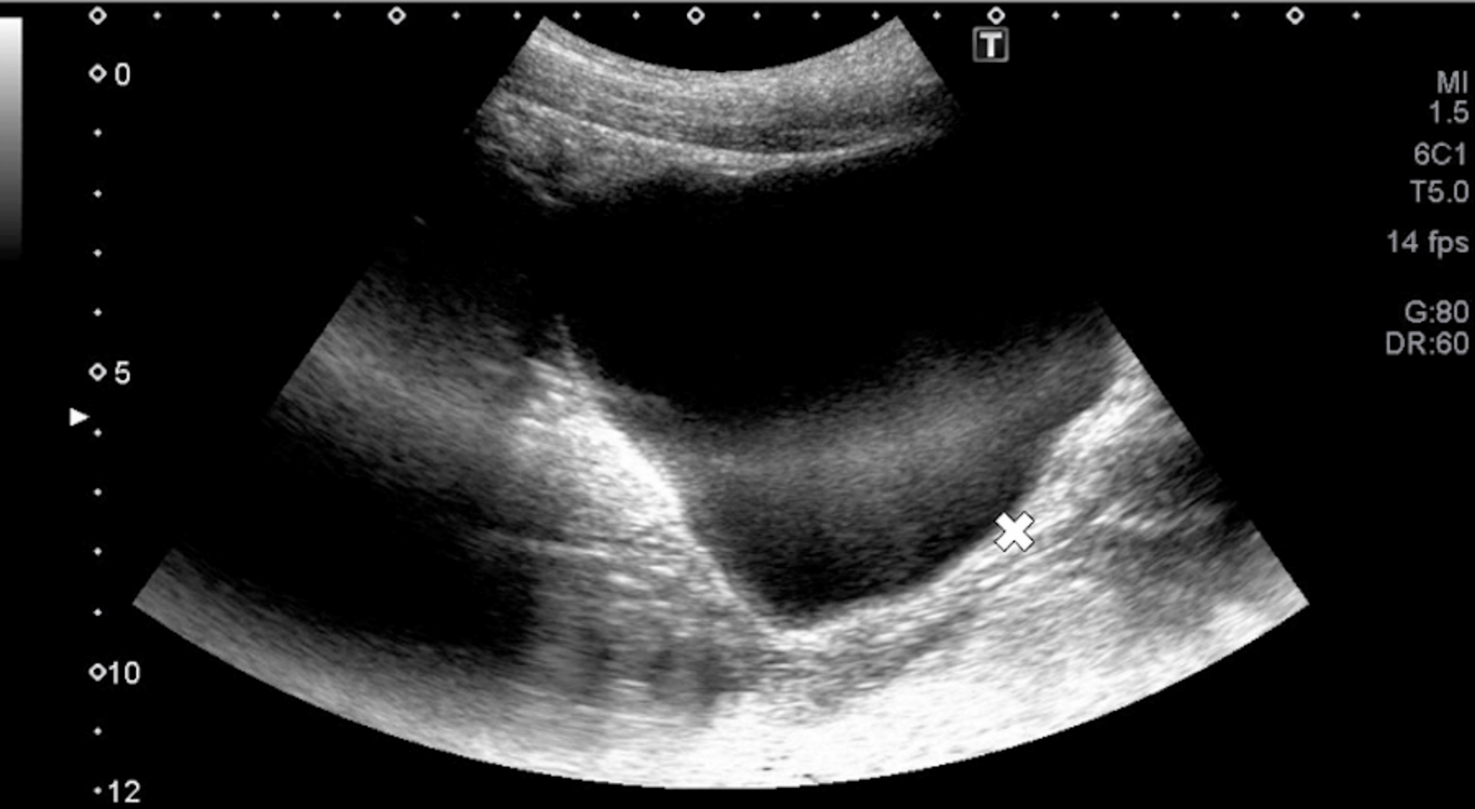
Ultrasound image of bladder base elevation at rest
